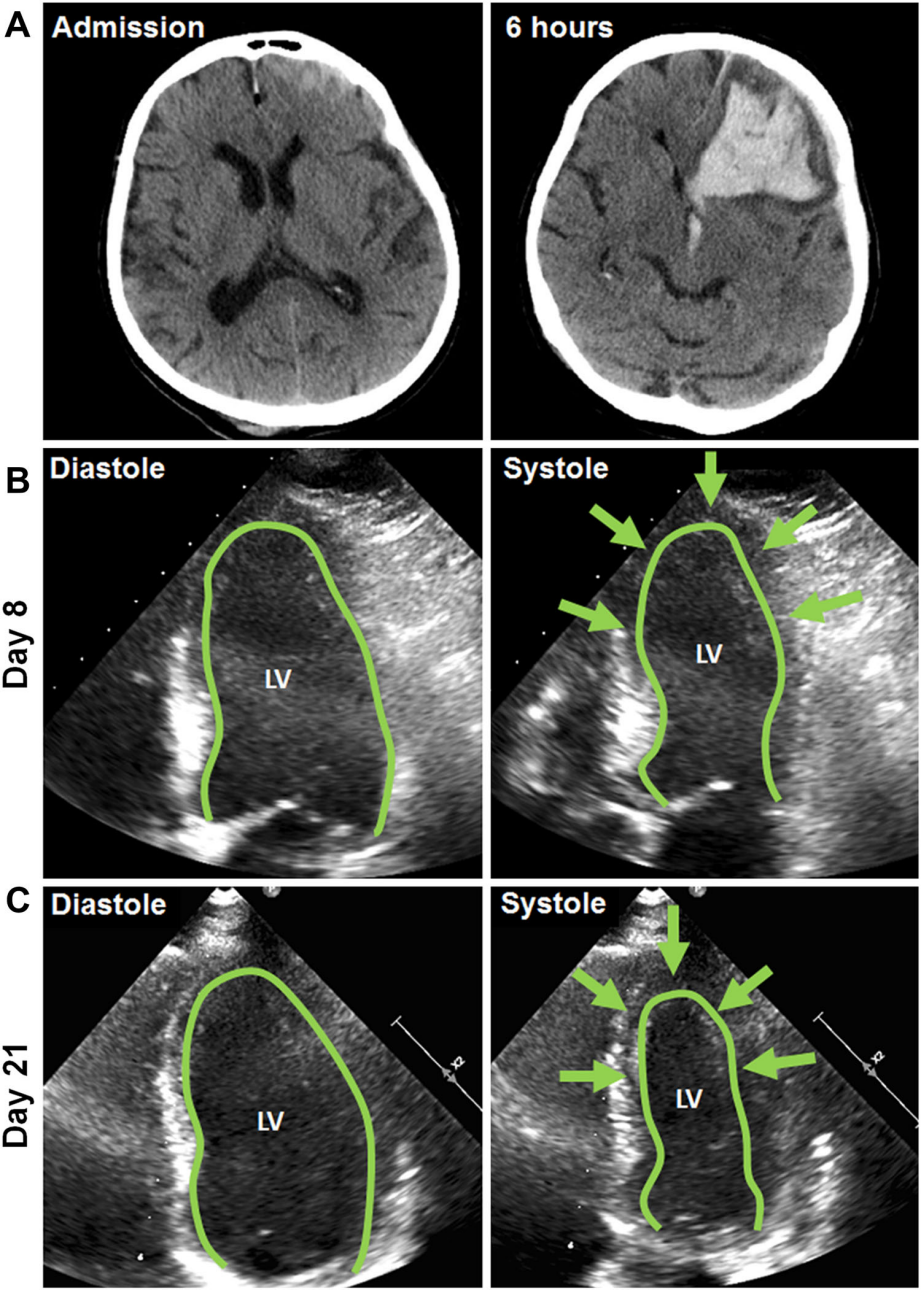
Neuroimaging and echocardiography *Panel A* indicate axial head computed tomography on admission and at follow-up 6 h later. Echocardiography on day 8 indicates moderate reduction in LVEF secondary to a persisting midventricular and apical hypo-/akinesia (*arrows*, *Panel B*). After 21 days (*Panel C*) LVEF nearly normalized and regional wall motion markedly improved (*arrows*) consistent with the typical presentation of a takotsubo cardiomyopathy. *LV* left ventricle, *EF* ejection fraction
